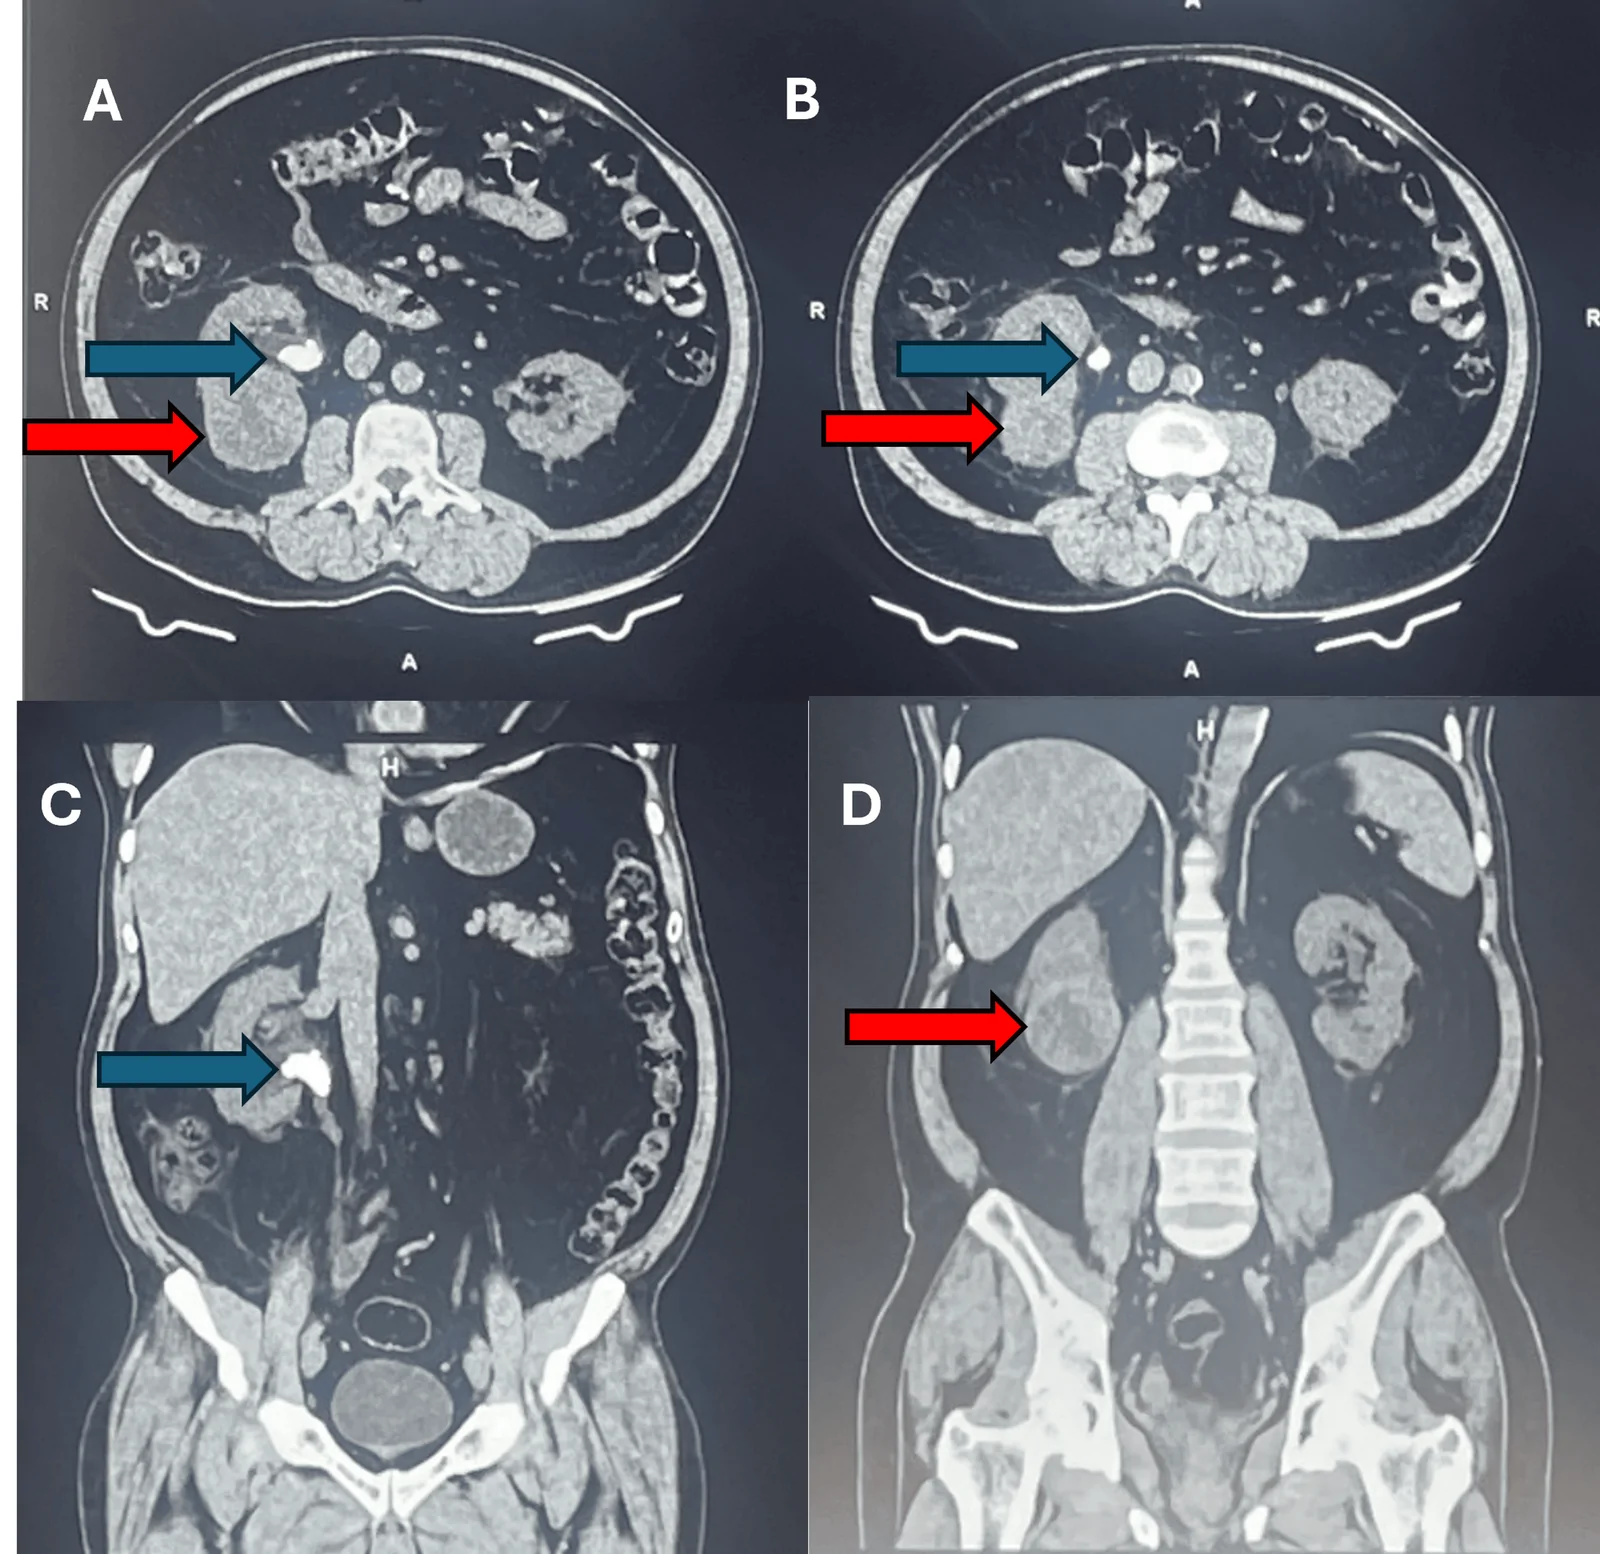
Noncontrast computed tomography of the abdomen showing right renal calculus and renal mass. (A, B) Axial sections. (C,D) Coronal sections. The blue arrow indicates calculus measuring 2.5 x 1.2 cm in the right renal pelvis extending into the right ureter. The orange arrow indicates a well-defined soft tissue mass in the lower pole of the right kidney.
